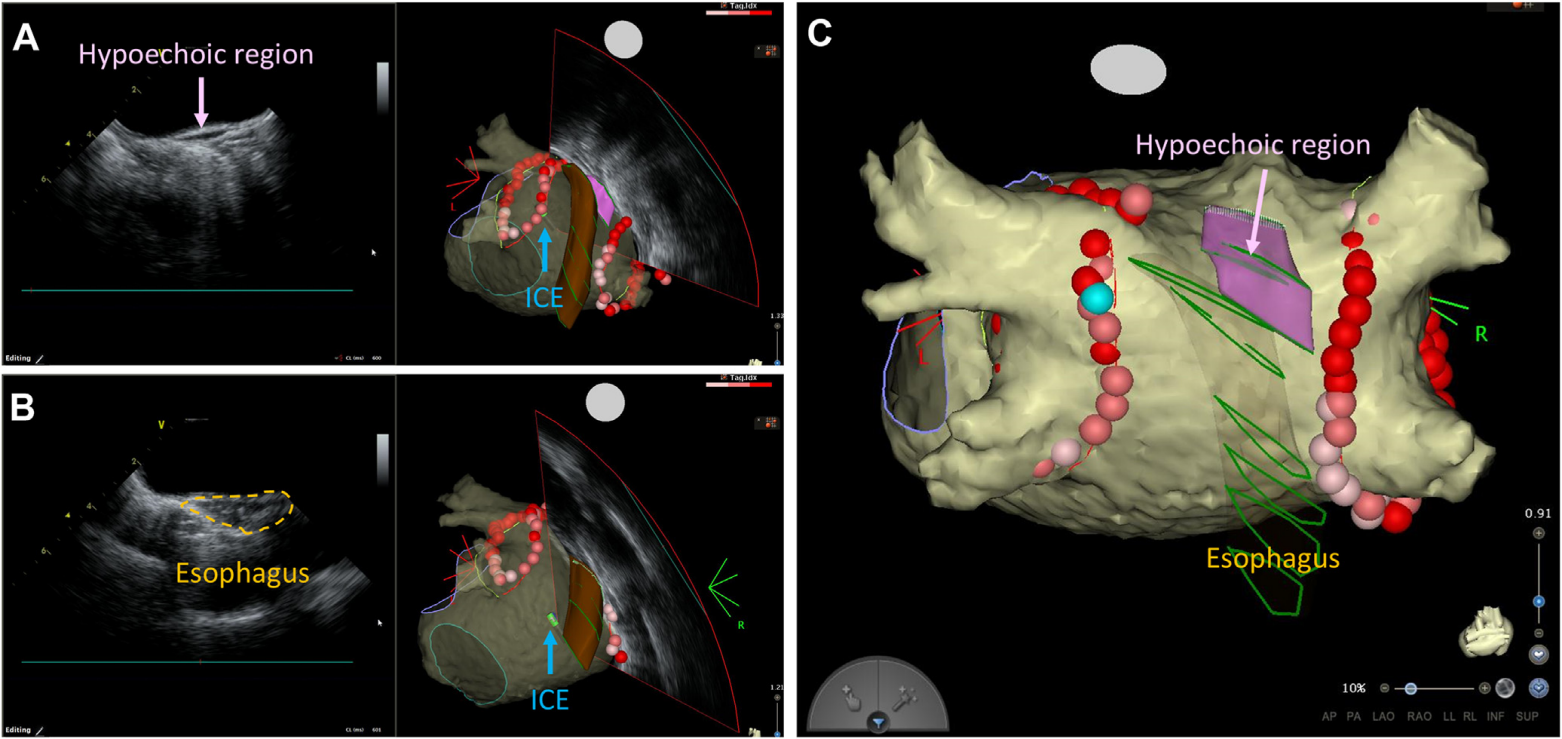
Visualization of hypoechoic regions on the LA roof using ICE. A: ICE image showing a hypoechoic region on the LA roof. The hypoechoic region is highlighted in *pink* on the CARTOSOUND map. B: ICE image depicting the esophagus on the LA posterior wall, marked in *brown* on the CARTOSOUND map. C: CARTOMerge image of the LA, showing the hypoechoic region suggestive of fat and the esophagus as identified using ICE. ICE = intracardiac echocardiography; LA = left atrium/atrial.
